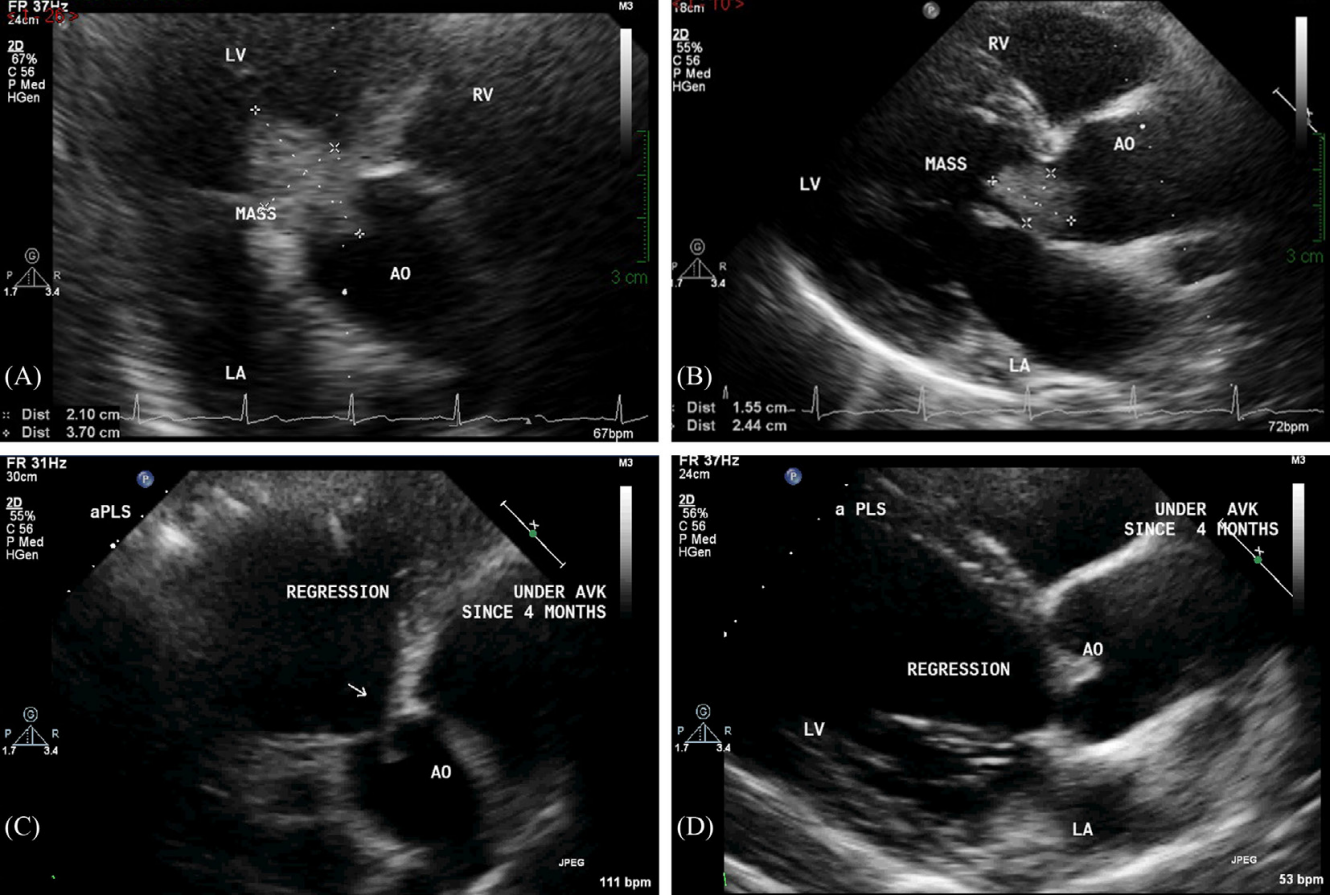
Transthoracic echocardiography performed at presentation reveals a large ovoid laminated mass on the aortic valve, measuring 3.7 cm × 2.1 cm on apical five chamber view (A) and 2.4 cm × 1.6 cm on parasternal short-axis view (B). Follow-up transthoracic echocardiography performed after 4 months of anticoagulation shows complete resolution of the valvular mass on apical five-chamber view (C) and parasternal short-axis view (D).
